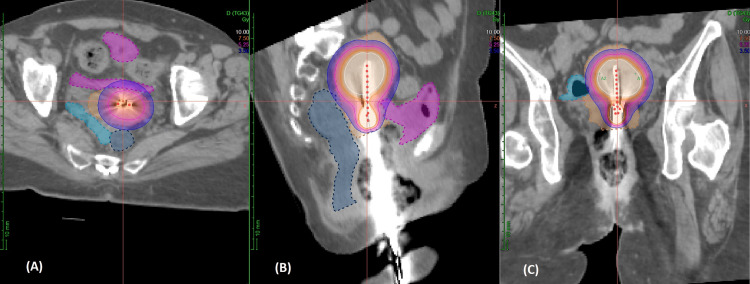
Axial (A), sagittal (B), and coronal (C) sections of CT-based planning for BT using the modified Rotte-Y applicator The prescription isodose (5.2Gy) line is pink, and the 3.5Gy isodose line is blue. The uterus and cervix are contoured in orange color. BT: Brachytherapy
